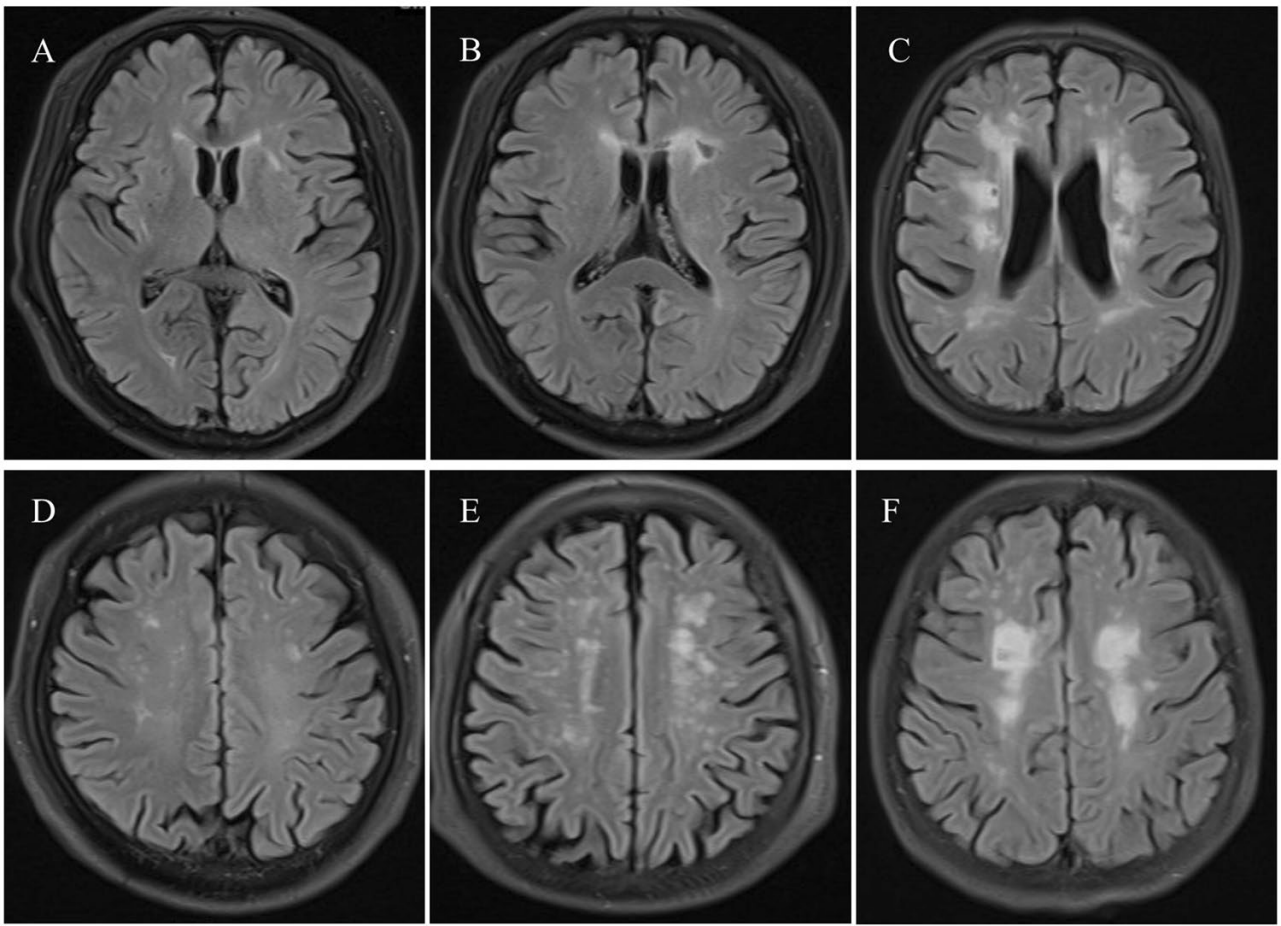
Differing severity of P-WMHs and D-WMHs. Magnetic resonance images showing severity in P-WMHs: (A) mild, (B) moderate and (C) severe. Magnetic resonance images showing severity in D-WMHs: (D) mild, (E) moderate and (F) severe
